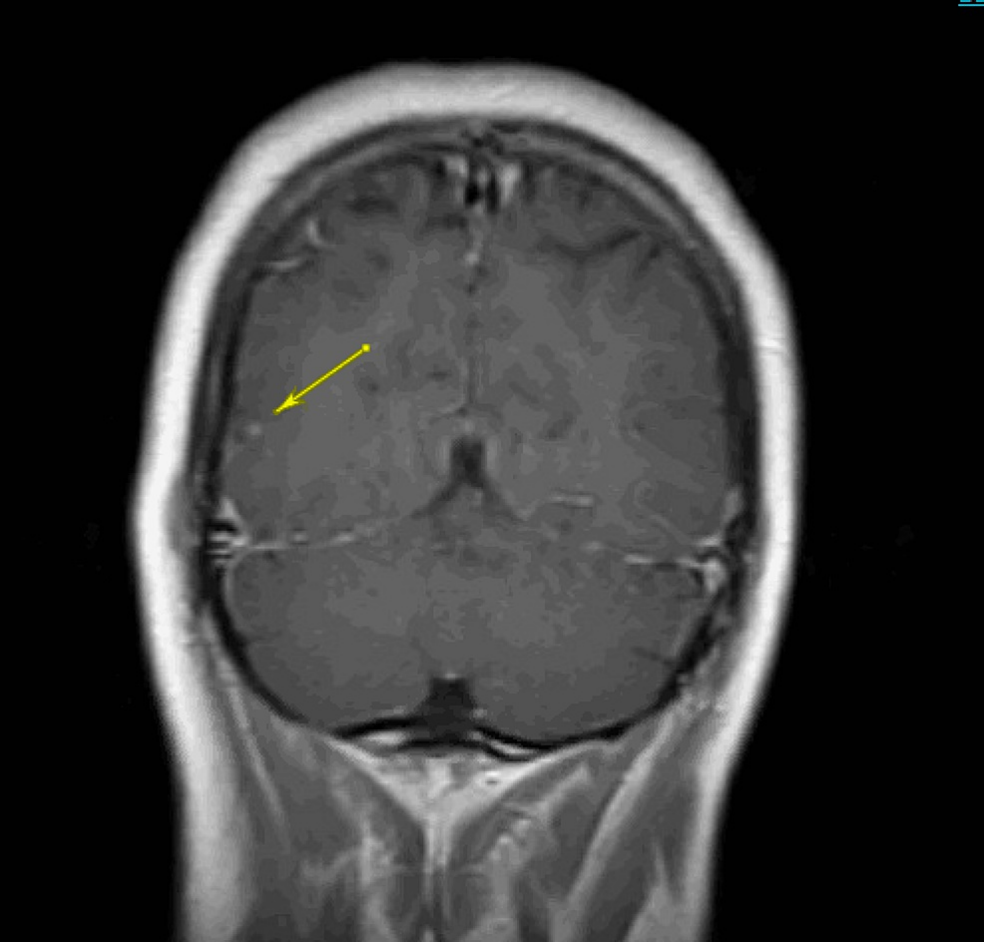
MRI Brain showing a tiny focus of enhancement in the right posterior temporal, parietal, and occipital areas.
